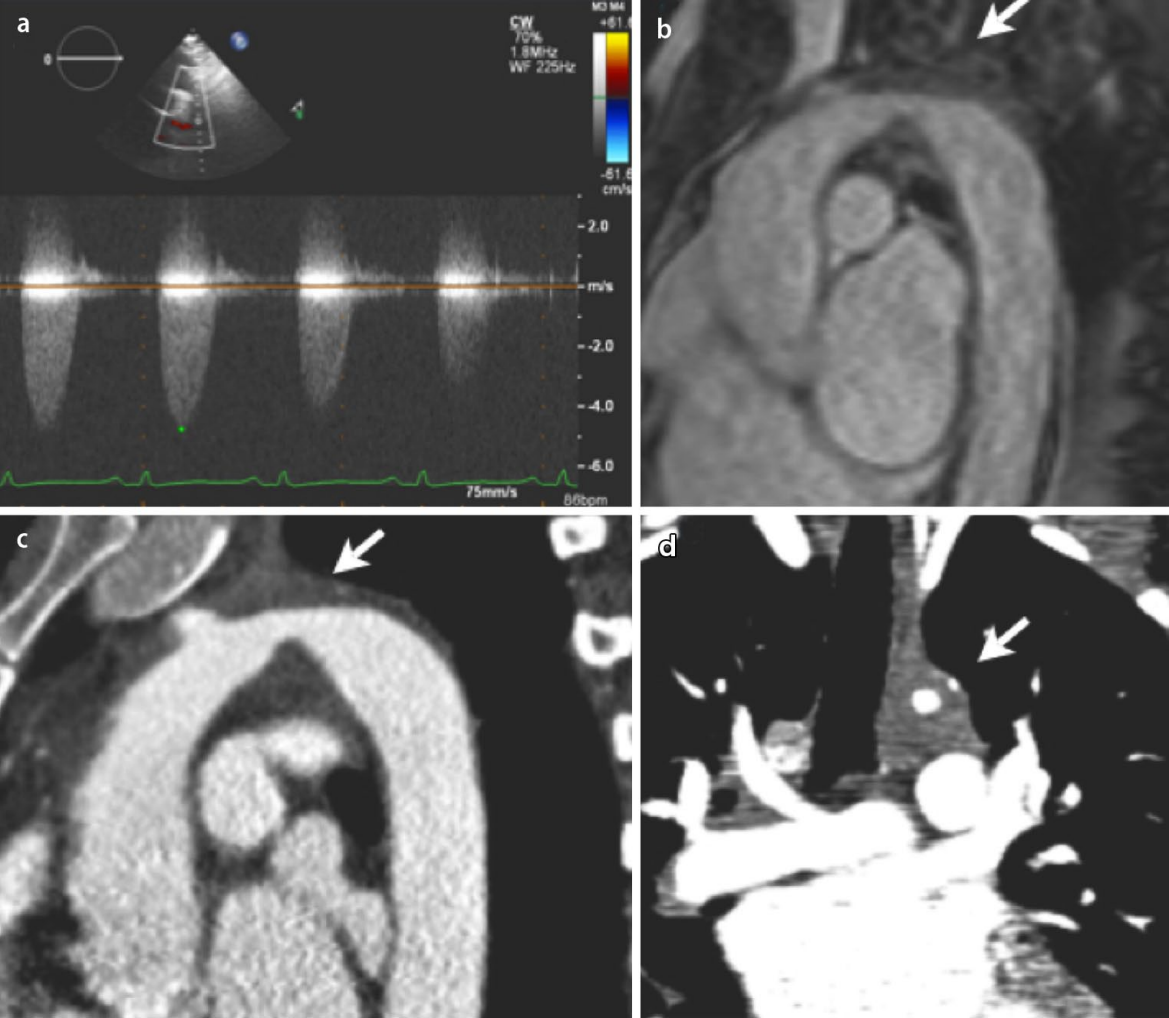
Imaging of the atypical aortic coarctation. a Doppler imaging of the descending aorta showing an elevated peak pressure gradient (90 mmHg). b Magnetic resonance and c, d computed tomographic images showing a coarction aorta on an atypical location in the aortic arch (*arrows*)
